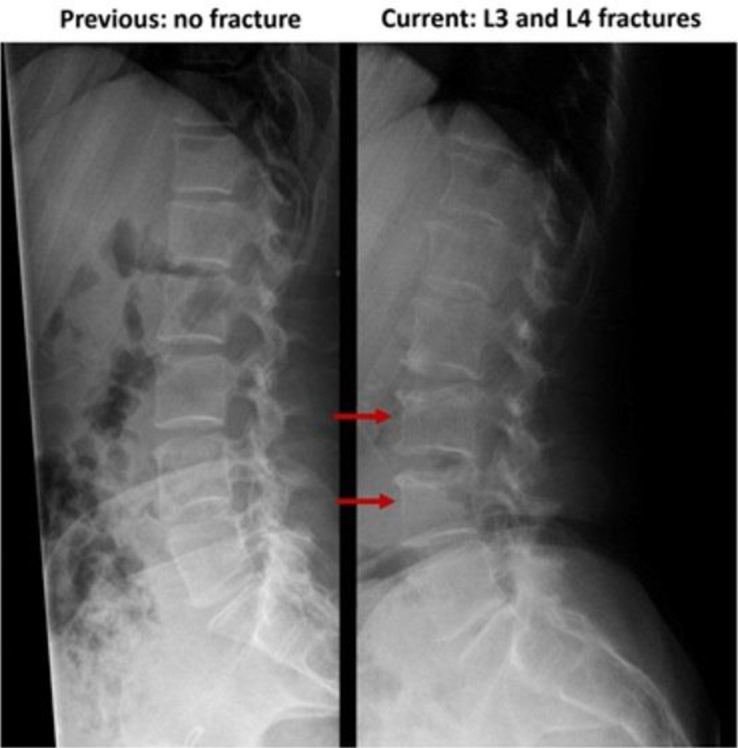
Lumbar spine X-rays, 10 years prior to and immediately following acute back pain.
